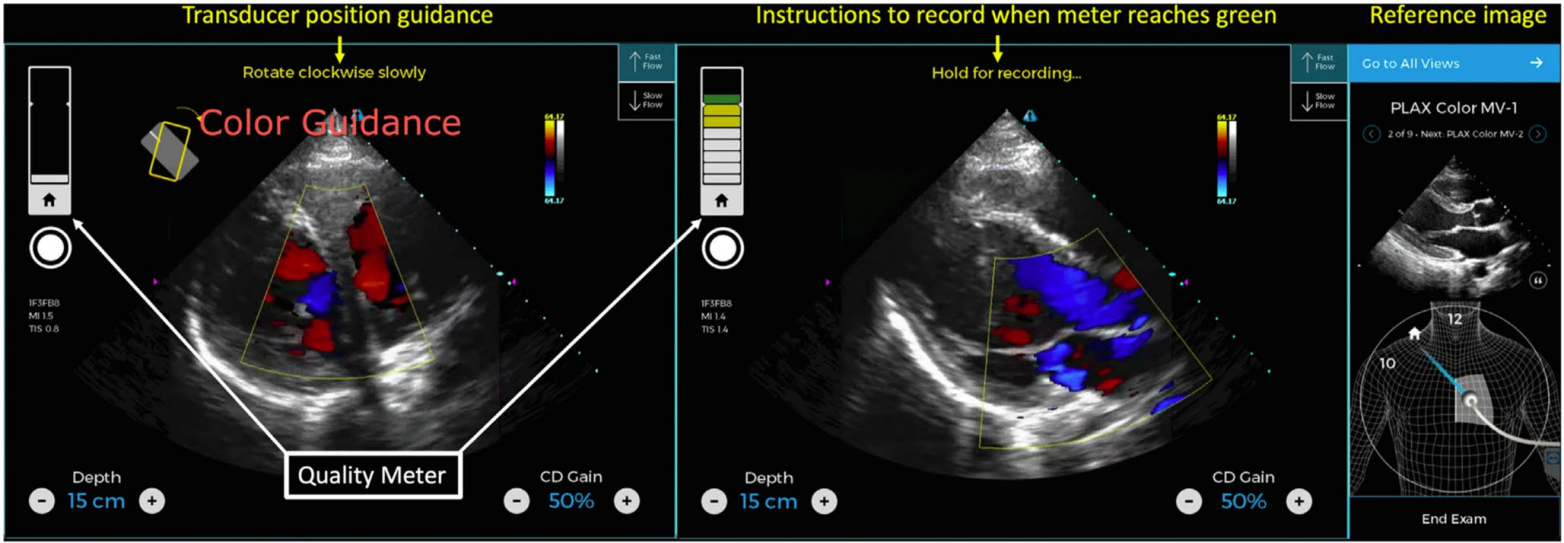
AI guidance user interface. Initial (*left*) and captured (*center*) PLAX color Doppler image of aortic outflow and mitral regurgitation using AI guidance. The reference image (*right*) instructs the novice where to place the transducer. Instructions for transducer movement result in an optimal image and an increase in the quality of the meter from white to yellow to green. Once the meter is in green, the scanner is instructed to manually acquire the image. Adapted from Peck et al. [14]
